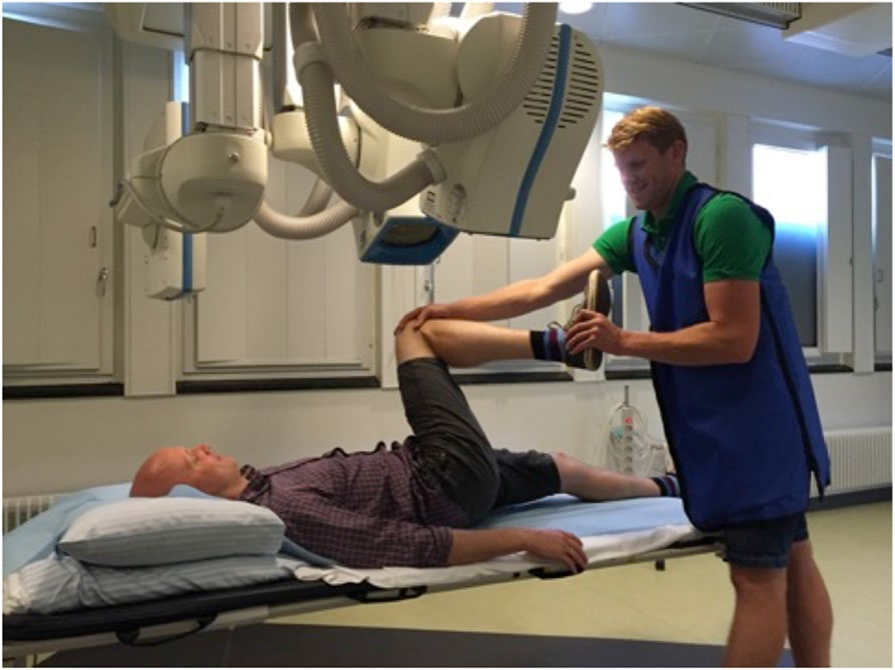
Clinical examination of patient during the FADIR test. The image also showing the radiographic setup
